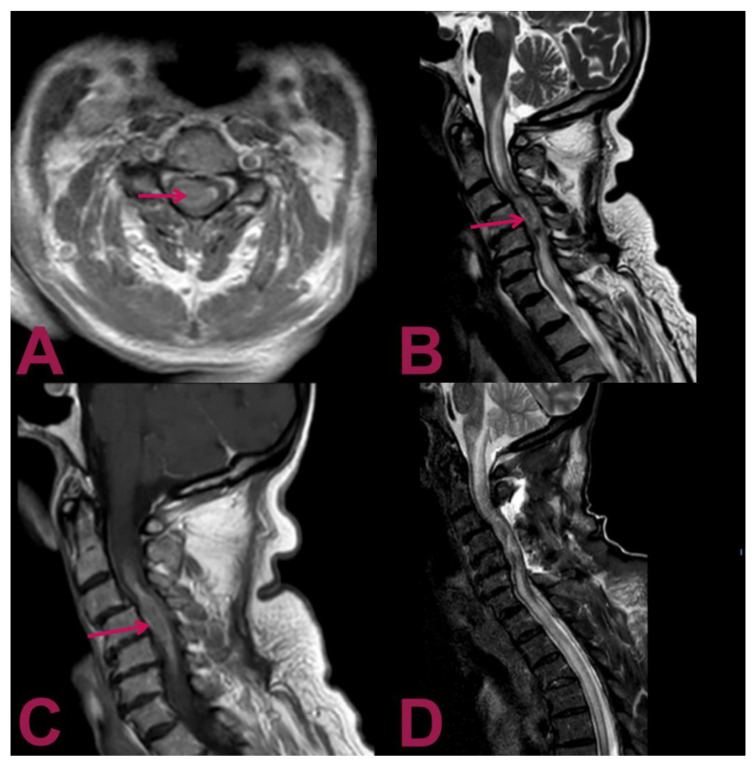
Magnetic resonance imaging (MRI) of the cervical spine. (A) Gadolinium-enhanced T1-weighted axial MRI shows an intra-axial lesion containing hemosiderin (arrow) with predominant right paramedian development. (B) T2-weighted sagittal MRI shows an extensive syringomyelic cavity, which covers the caudal portion of the floor of the IV ventricle and affects the posterior portion of the bulb–medullar junction extending up to the D5 level inferiorly. The syringomyelic cavity is the result of the newly formed tissue extending from C4 level for about 6 cm with a maximum diameter of about 15 mm (arrow). (C) Gadolinium-enhanced T1-weighted sagittal MRI displays the intramedullary mass at the C2–C6 level with a heterogeneous contrast enhancement pattern (arrow). (D) Postoperative T2-weighted sagittal MRI reveals the outcomes of hemilaminectomy C3–C4–C5 and cervical myelotomy with partial removal of the lesion; noticeably, despite the invasive nature of the surgical intervention, the previous parameters have not deteriorated; no worsening in the syringomyelic cavity is present, and the peri-wound oedema is not increased.
